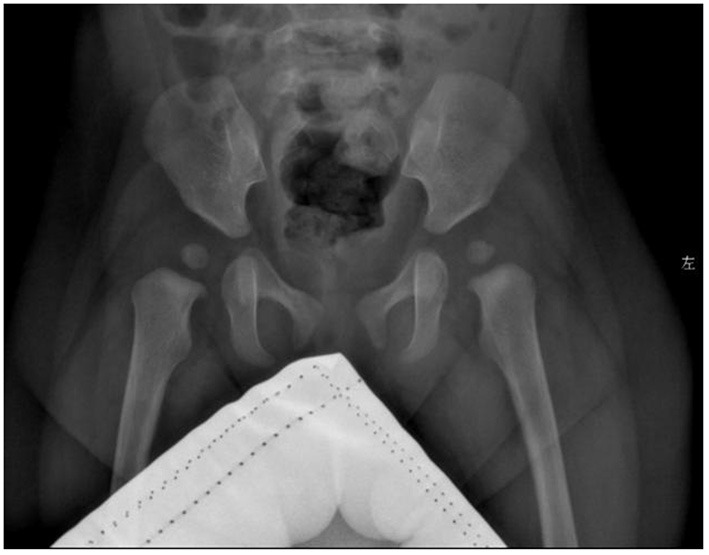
Radiographs of the child's hip joint show slightly flat and shallow bilateral acetabular fossa and enlarged bilateral acetabular angle.
